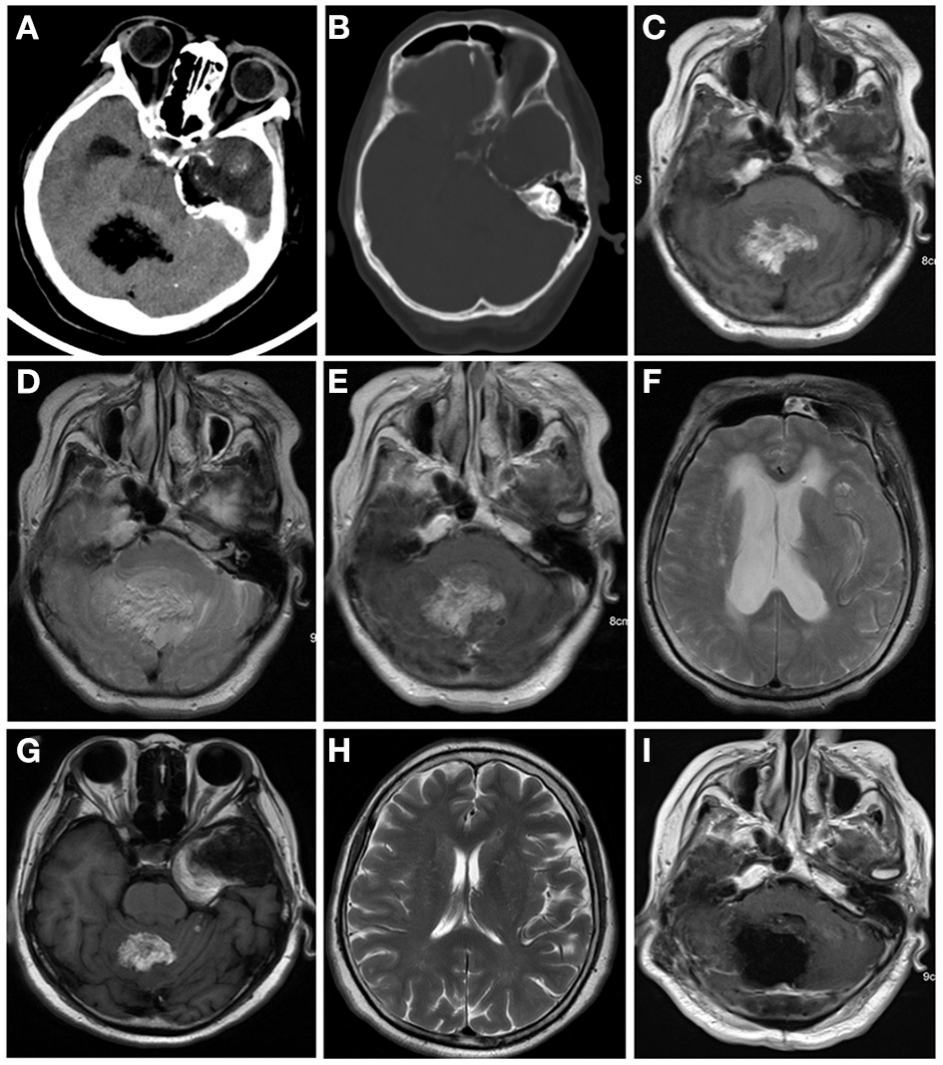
Computed tomography (CT) and contrast-enhanced magnetic resonance imaging (MRI) were performed in June 2018 (A–E). CT images of brain window images demonstrated a well-demarcated mass with fat density in the left temporal lobe and cerebellar vermis with calcification (A,B). MRI revealed a hyper- and isointense mixed mass on T1-weighted image (C) and iso- and hyperintense mixed mass on T2-weighted image (D). Gadolinium-enhanced T1-weighted image showed heterogeneous enhancement (E). The lesion in the cerebellum compressed the fourth ventricle (E) and caused supratentorial obstructive hydrocephalus (F). In 2013, the cerebellum lesion was relatively small (G) and did not cause obstructive hydrocephalus (H). Postoperative MRI indicated the cerebellar mass was near-totally removed (I).
